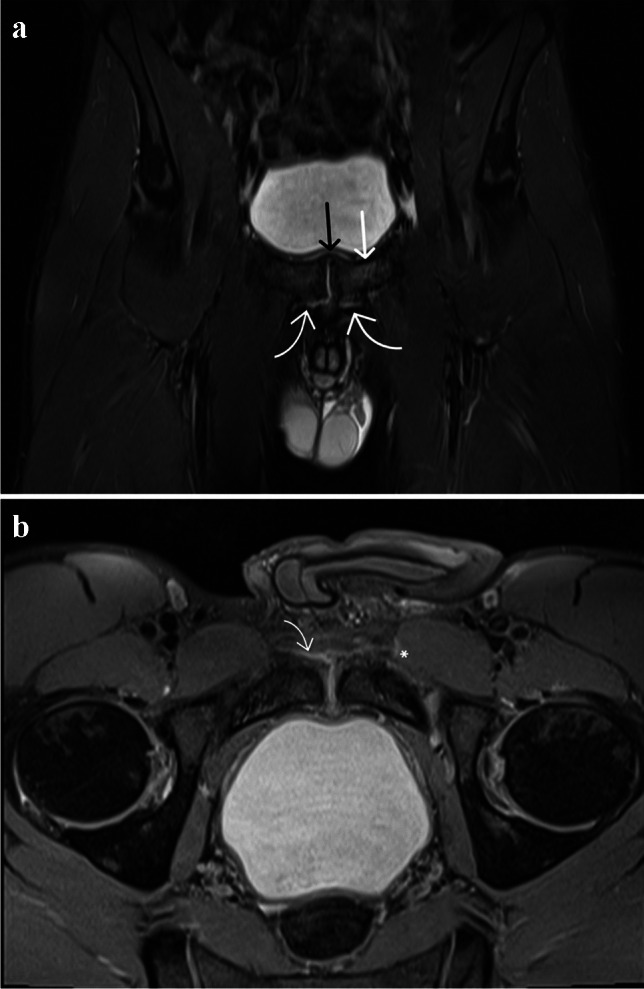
Symptomatic 27-year-old male athlete. a coronal STIR and b axial oblique PD-weighted fat suppressed MR images show left-sided pubic body marrow oedema scored as grade 2 (white straight arrow), bilateral capsule/aponeurosis tears (curved arrows) and left capsule/aponeurosis soft tissue oedema (asterisk). Right capsule/aponeurosis soft tissue oedema was present but not shown on above images. The right pubic body marrow signal was scored grade 1, therefore categorised as normal. Symphysis pubis superior bone spurring (black arrow) scored as present
